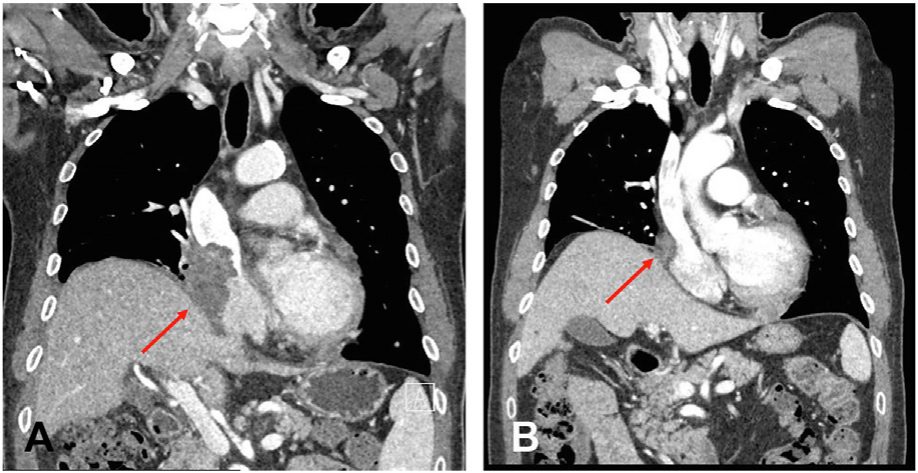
Computed tomography scan showing malignant mesothelioma. Computed tomography findings of a malignant pericardial mesothelioma (A) before chemotherapy (*arrow*) and (B) after 10 chemotherapy cycles (*arrow*).
